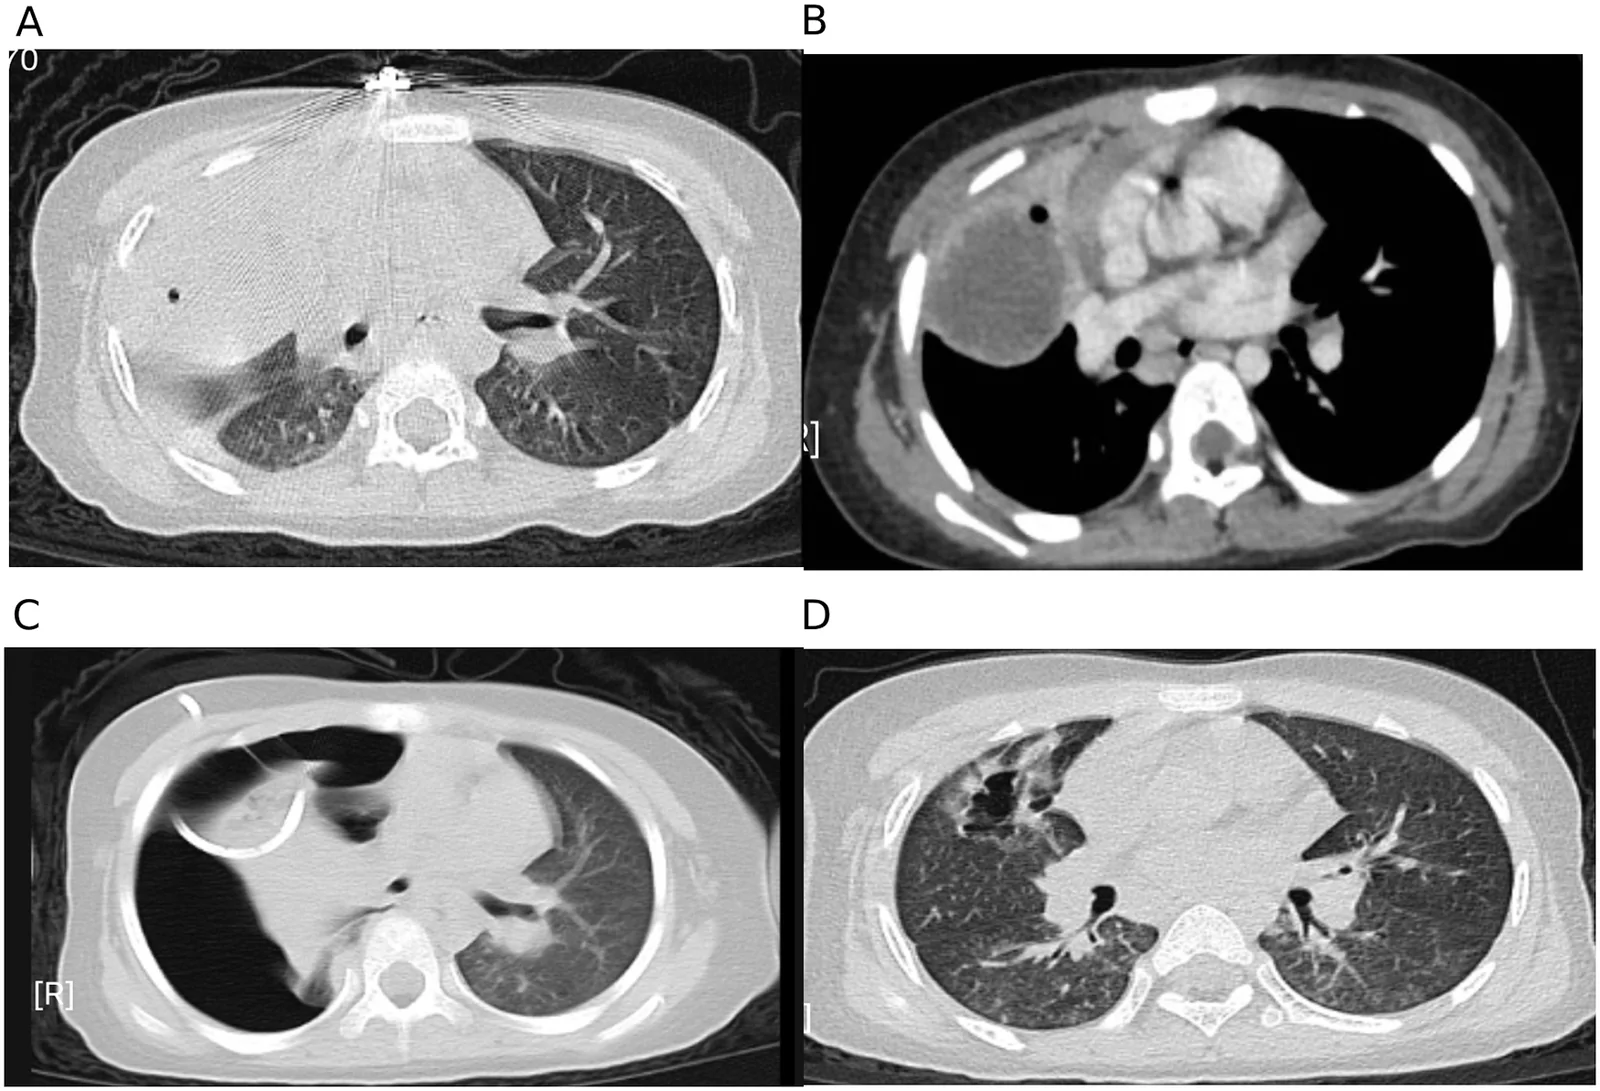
Serial chest CT images of the patient. (A) Chest CT images obtained at presentation demonstrate a large patchy high-density opacity (68 mm × 46 mm) in the right upper lobe with a small pleural effusion. (B) Contrast-enhanced chest CT images obtained after 11 days of treatment demonstrate partial resolution of the right upper lobe mass-like opacity (58 mm × 39 mm). (C) Pneumothorax on day 5 after ultrasound-guided percutaneous drainage. (D) Follow-up chest CT images obtained 3 months after discharge show a cavity (22 mm × 15 mm) along the right horizontal fissure.
